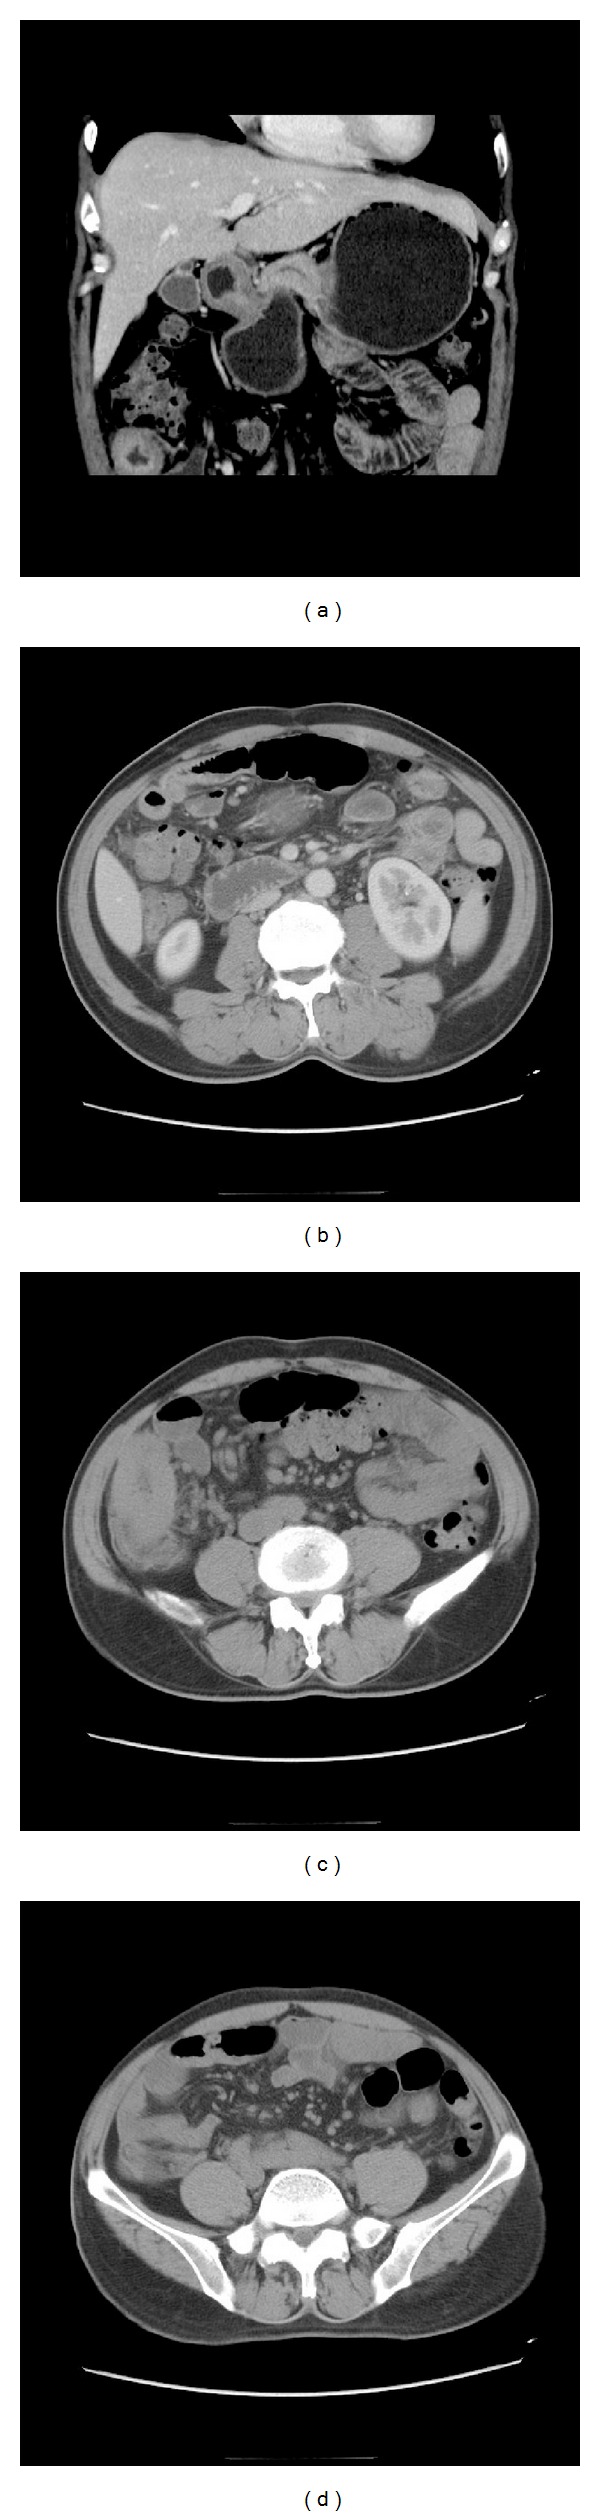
HRCT scan of the abdomen showed severe segmental narrowing, a cobblestone appearance of the terminal ileum, small-bowel wall thickening, and mesenteric adenopathy.
